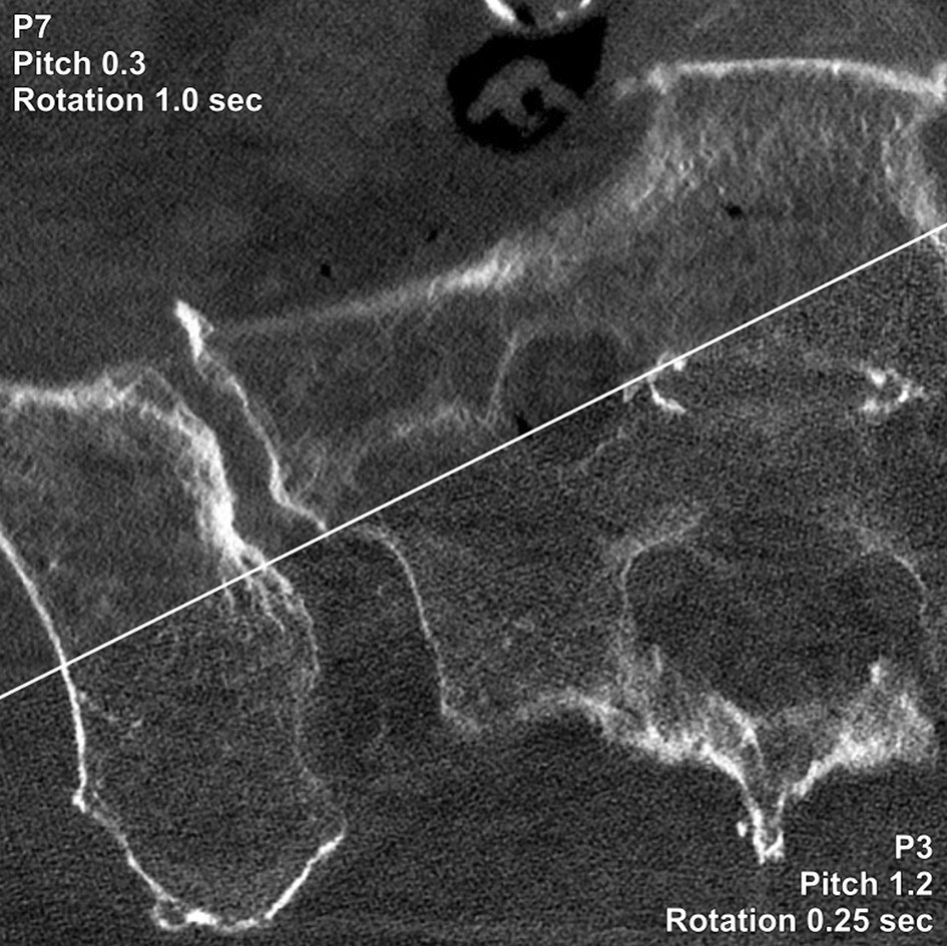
Image quality comparison between a low pitch/slow gantry rotation (P7) and high pitch/fast gantry rotation (P3) protocol in a pelvic photon-counting CT scan. Scan duration (P7: 35.6 vs. P3: 3.8 s) and raw data file size (191.4 vs. 12.8 GByte) differed substantially, whereas CTDI_vol_ was identical (5.83 vs. 5.83 mGy).
